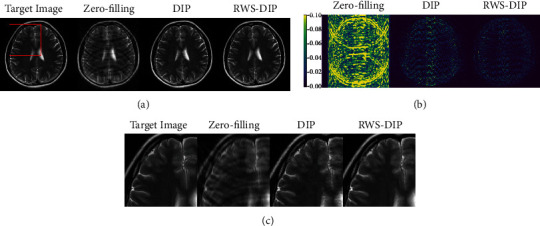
Comparison of reconstructions of the target MR image in Brain B using Cartesian undersampled mask with 30% sampling rate: (a) the target image and reconstruction results, (b) the corresponding error images, and (c) the corresponding zoom-in images.
